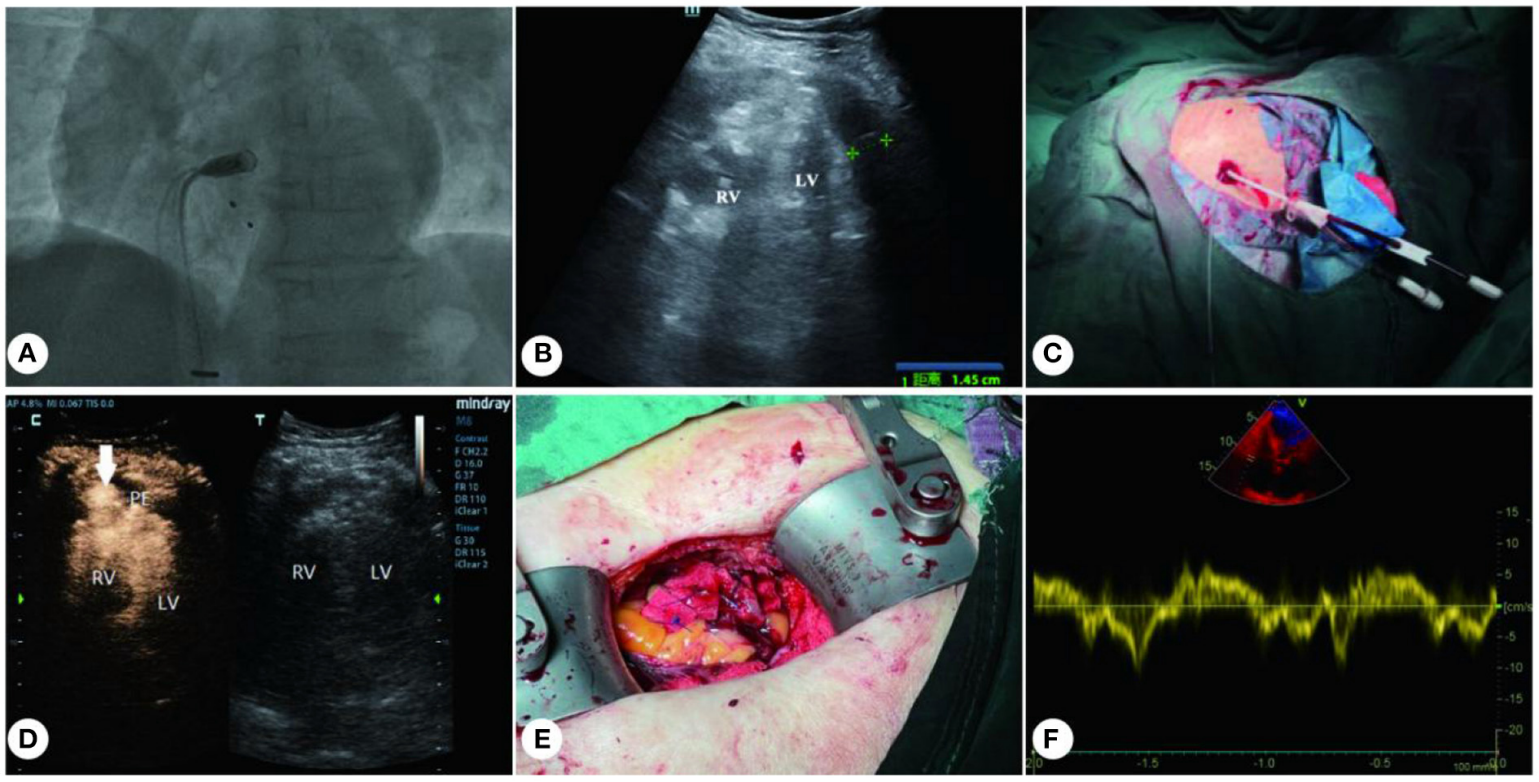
Treatment for iatrogenic right ventricular perforation. (A) Delivery system carrying the MLP to right ventricle. (B) Medium-volume effusion in the pericardial cavity. (C) 12F pigtail catheter placed (under ultrasound guidance) into the pericardial cavity. (D) CEUS shows right ventricular free wall perforation (with arrow indication). (E) 3-0 prolene with spacers were used to close the RV perforation. (F) Recovery of cardiac function after surgery. RV, right ventricle; LV, left ventricle; PE, pericardial effusion.
